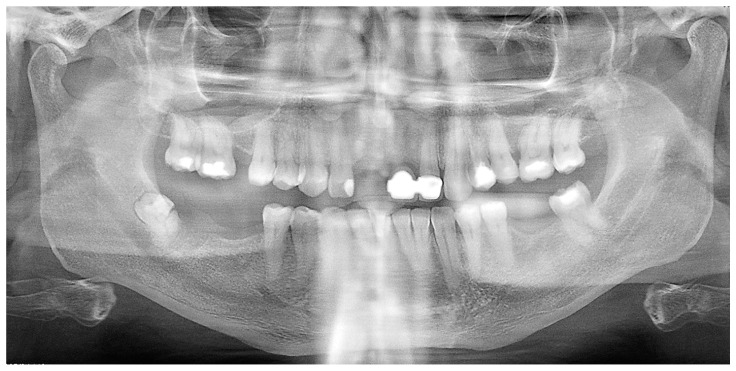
Orthopantomogram (OPT) confirming a semi-impacted fractured wisdom tooth on the right side of the jaw.
